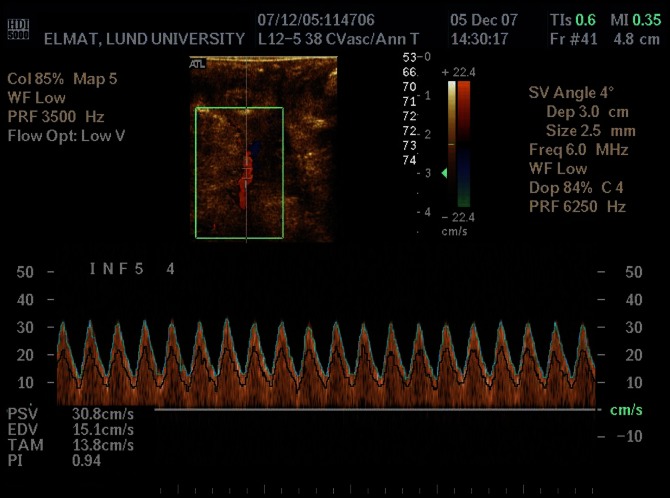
Doppler signals of blood velocity recorded from the umbilical artery of a fetal lamb. Within the Doppler spectrum displayed on the screen of the ultrasound scanner, the on-line estimated maximum velocity and the mean weighted velocity are displayed as a light blue and a black line, respectively. The automatically calculated pulsatility index (PI) and time-average velocity (TAM) values are shown in the lower left corner. The waveform of the blood velocities with positive signals throughout the heart cycle indicate a low resistance to flow in the placenta, i.e. a normal fetoplacental blood flow.
